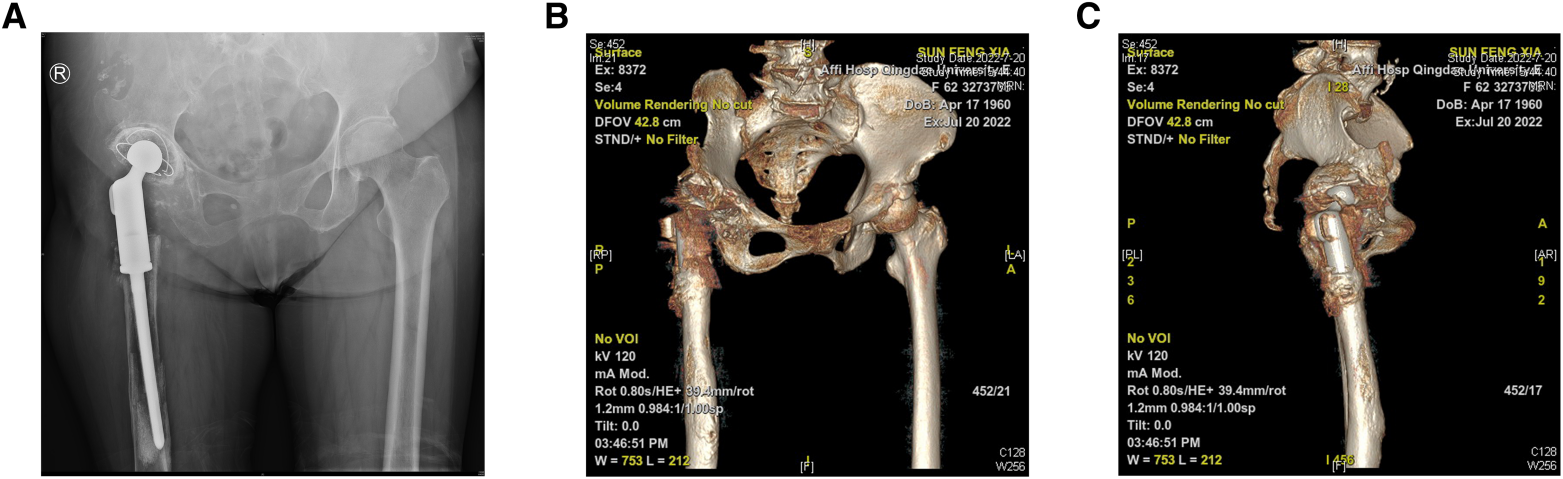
(A) A pelvic radiograph (anteroposterior view) shows the right acetabular prosthesis moving up significantly and the right femoral prosthesis sinking, with a slight and massive osseous defects on the pubis and proximal femur, respectively. (B,C) Three-dimensional computed tomography reconstruction reveals the abnormal position of the prosthesis and osseous defects.
